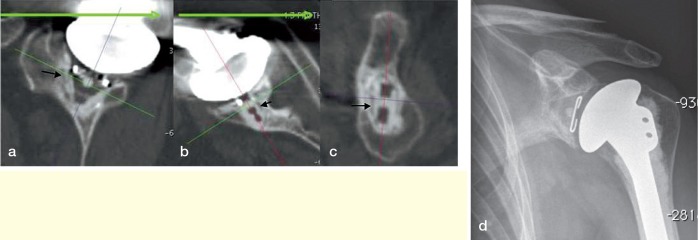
Radiolucent line at the cement-bone interface (black arrows) visualized by using the described protocol, in the sagittal plane of the glenoid implant (a), in the axial plane of the middle part of the implant (b), in the coronal plane of the implant, passing through the keel hole (c). Plain radiograph of the same shoulder for comparison (d).
